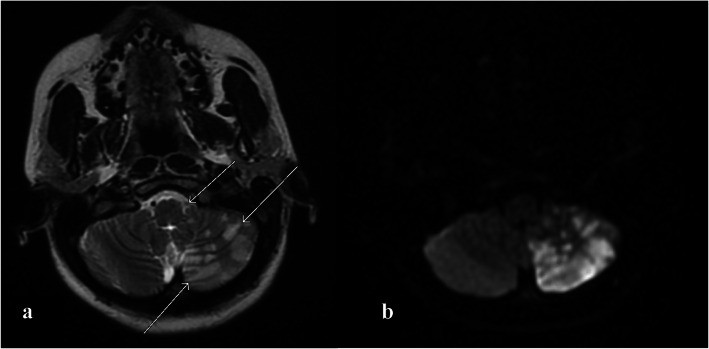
a. T2-weighted MRI of the brain demonstrates an extensive acute infarction of the left cerebellar hemisphere in the PICA territory and a left vertebral artery occlusion. b. Diffusion weighted MRI of the brain further confirms the extensive acute infarction of the left cerebellar hemisphere
